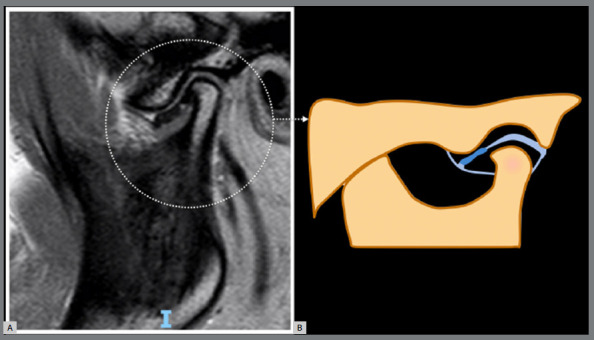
Hypertrophic phenotype: a marginal hypertrophy with sclerotic borders and exophytic angular formation of osseous tissue arising from the surface of the condyle (osteophyte) can be observed. A) Referential temporomandibular joint MRI sagittal proton density image. B) Temporomandibular joint scheme. Adapted from ROAMES - Rapid OsteoArthritis MRI Eligibility Score (Source: Roemer et al.^8^, 2020).
